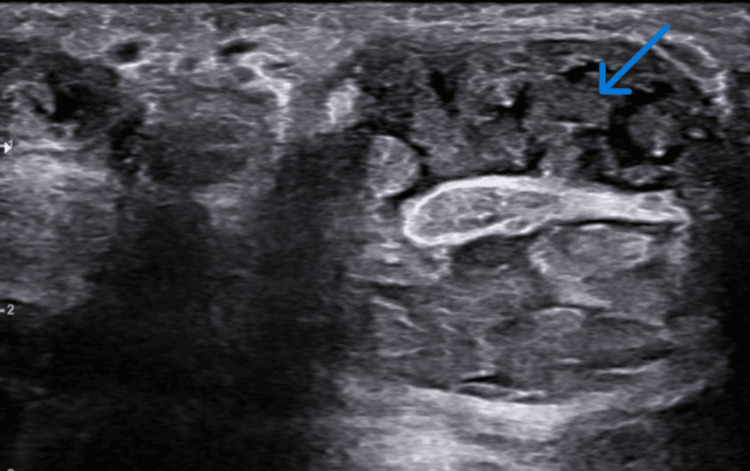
Ultrasound scan demonstrating multiple, slightly echogenic nodules with intervening fluid at the volar aspect of the hand
